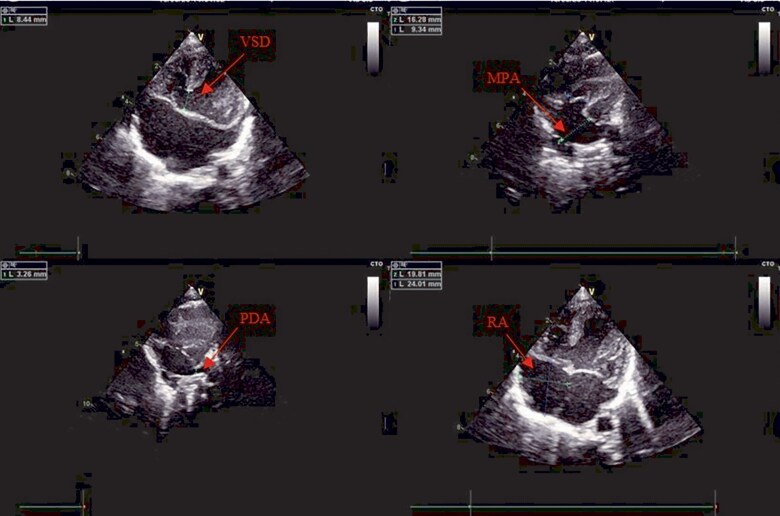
Preoperative echocardiogram. Note: VSD = ventricular septal defect. PDA = patent ductus arteriosus; MPA = main pulmonary artery; RA = right atrium.
